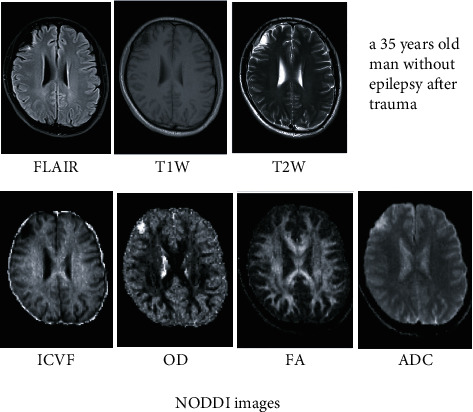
A 35-year-old man without epilepsy one year after trauma. He had softening foci with FLAIR hyperintense lesions in the right frontal lobe (FLAIR, T1W and T2W images, and NODDI ICVF, OD, FA, and ADC images).
